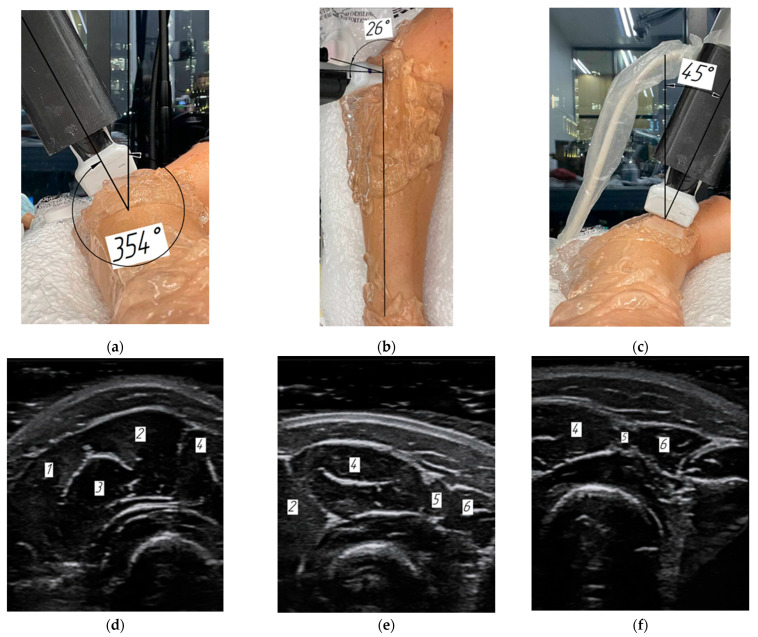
Ultrasound sensor position at angles to the vertical axis (a–c) and the corresponding ultrasound images (d–f): (a,d)—at an angle of 45 degrees to the vertical axis in the projection of muscles 1—m. brachioradialis, 2—m. extensors carpi radiales longus, 3—m. extensors carpi radiales brevis, 4—m. extensor digitorum; (b,e) at an angle of 26 degrees to the axis in the projection of muscles 2—m. extensors carpi radiales longus, 4—m. extensor digitorum, 5—m. extensor digiti minimi, 6—m. extensor carpi ulnaris; (c,f)—at an angle of 354 degrees to the axis in the projection of muscles 4—m. extensor digitorum, 5—m. extensor digiti minimi, 6—m. extensor carpi ulnaris.
